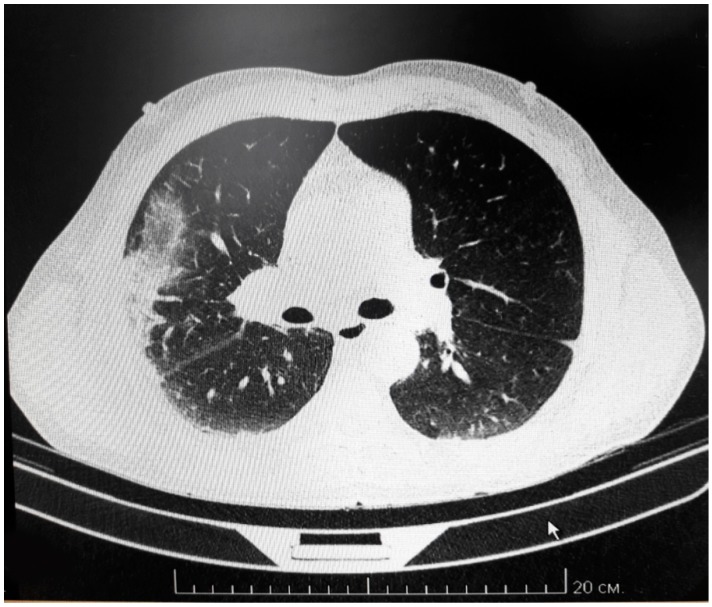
Multi-slice CT of fatal HFRS lung.
